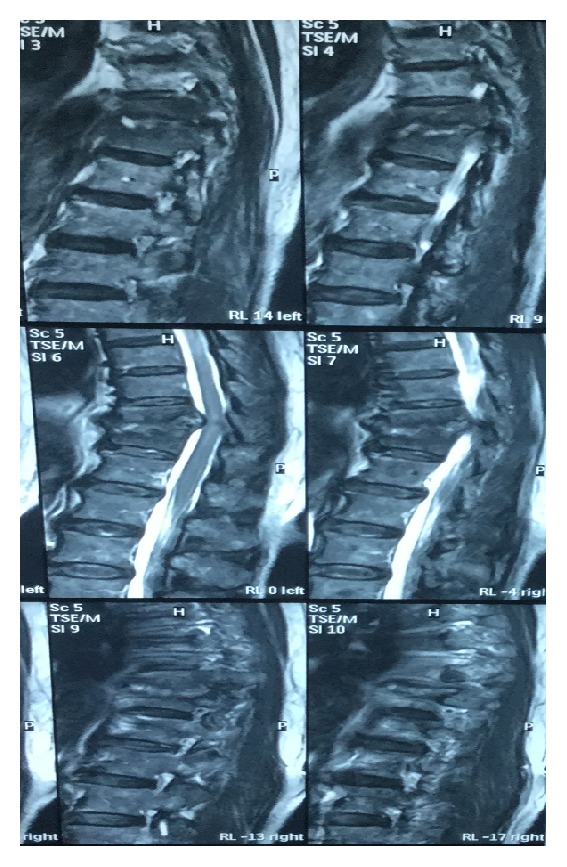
MRI thoracic spine T10-T11 discitis with minimal soft-tissue component and vertebral body.
